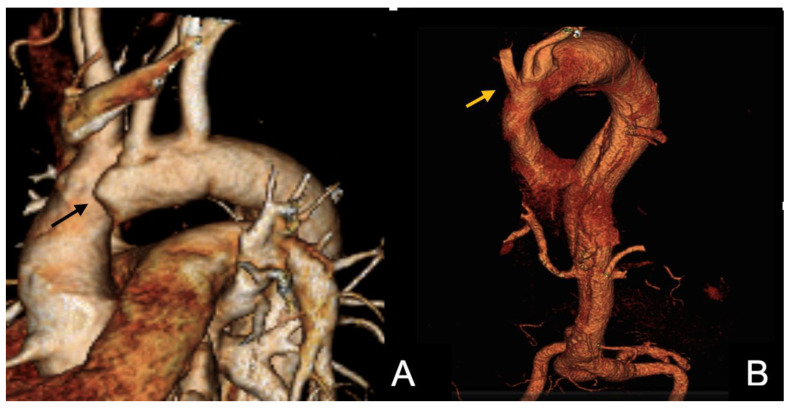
volume rendering of the baseline computed tomography angiography (CTA) of two patients included in this series. The arrows indicate the primary entry tear in Ishimaru zone 0 (A) and zone 1 (B).
